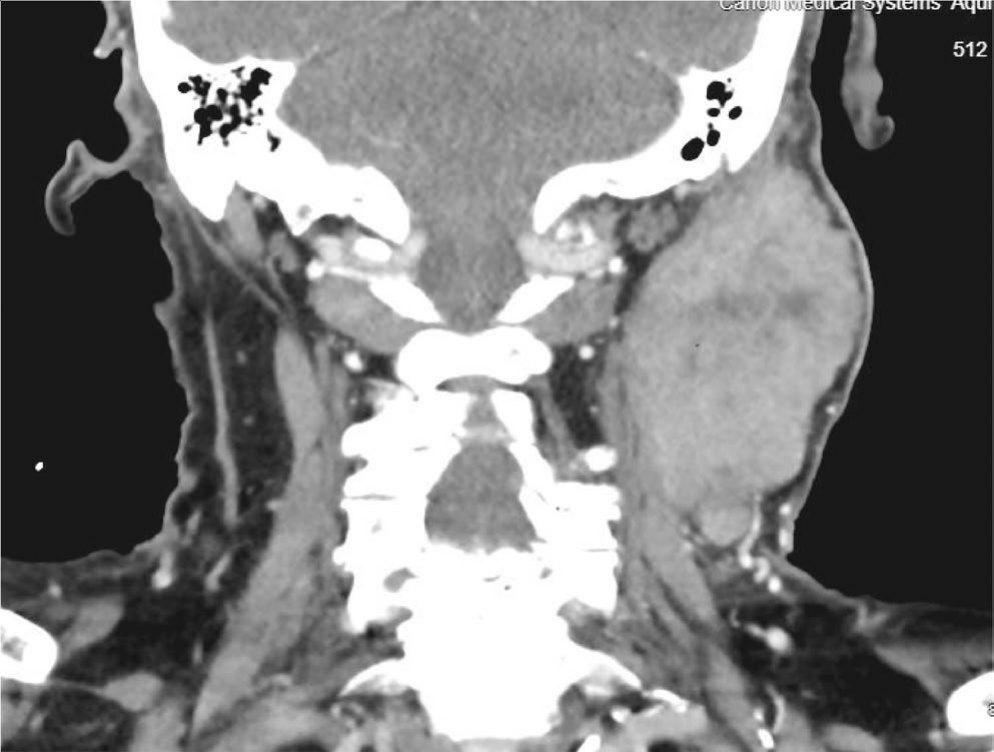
CT neck scan one month prior to clinic presentation.
